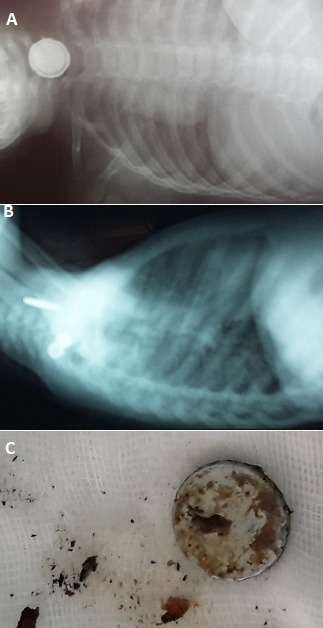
A) radiographie thoracique de face montrant l'opacité ronde cervicale; B) radiographie thoracique de profil montrant l'opacité linéaire postérieure par rapport aux clartés de la trachée; C) pile bouton après extraction
